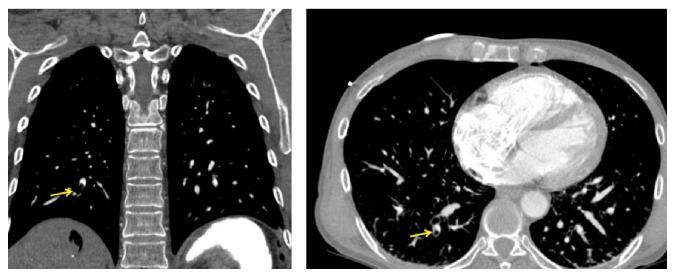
Contrast enhanced coronal and axial chest CT. Subsegmental artery air embolism in apical segment of right lower lobe surrounded by contrast media is observed (yellow arrows).
